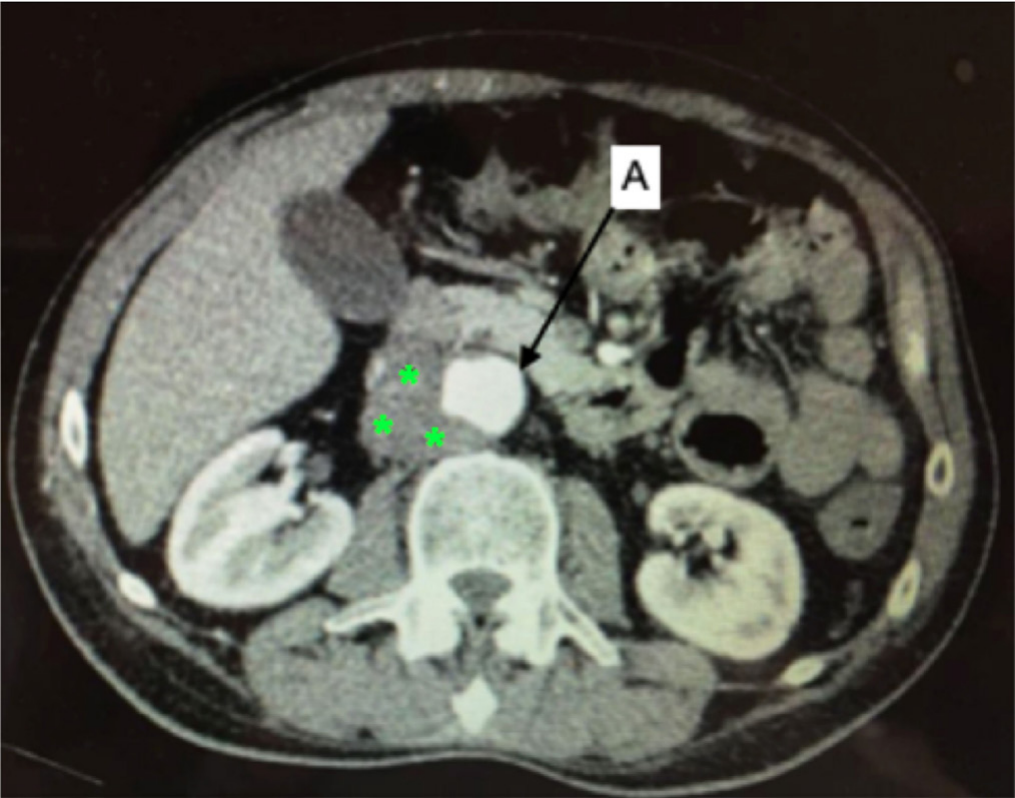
Computed tomography with intravenous contrast, axial slice. (A) Aorta; the asterisks mark an image compatible with periaortic collection/mass.
